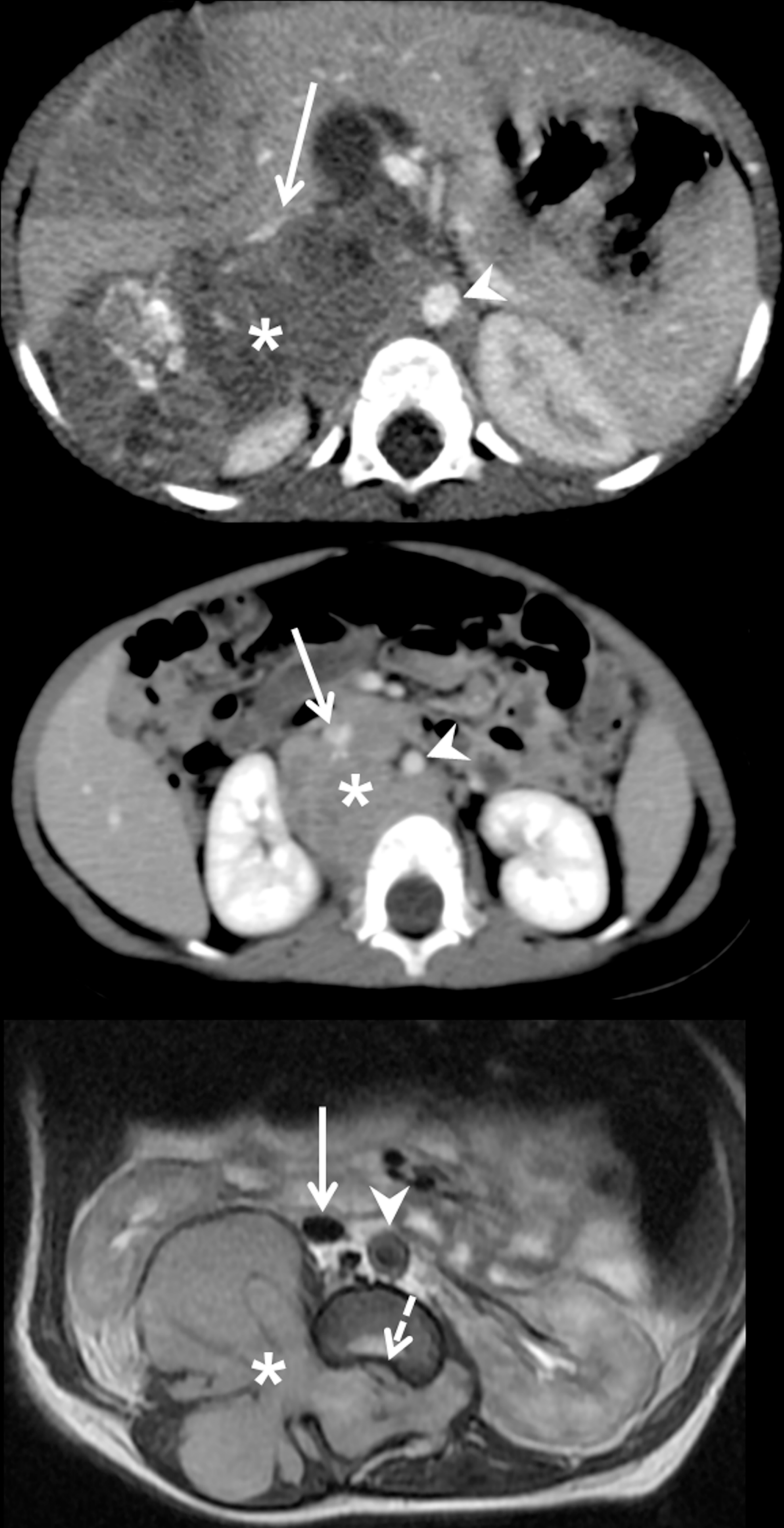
Imaging phenotypes of abdominal neuroblastomas. (a) 18-month-old girl with M-stage right adrenal MNA neuroblastoma. Enhanced CT scan at diagnosis. The primary tumor (*) is centered on the right adrenal gland between the right kidney and the inferior vena cava (arrow) and extends medially in contact with the aorta (arrowhead). (b) 12-month-old girl with M-stage retroperitoneal periarterial SCA neuroblastoma. Enhanced CT scan at diagnosis. The primary tumor (*) is centered in the median retroperitoneum around the aorta (arrowhead) and behind the inferior vena cava (arrow). (c) Newborn with L2-stage lumbar dumbbell paravertebral NCA neuroblastoma. Axial T2-weighted MRI at diagnosis. The primary tumor (*) is centered on the right paravertebral chain and invades the psoas and spinal muscles and fills the spinal canal, compressing the spinal cord (dotted arrow). The tumor is totally separated from the inferior vena cava (arrow) and the aorta (arrowhead).
